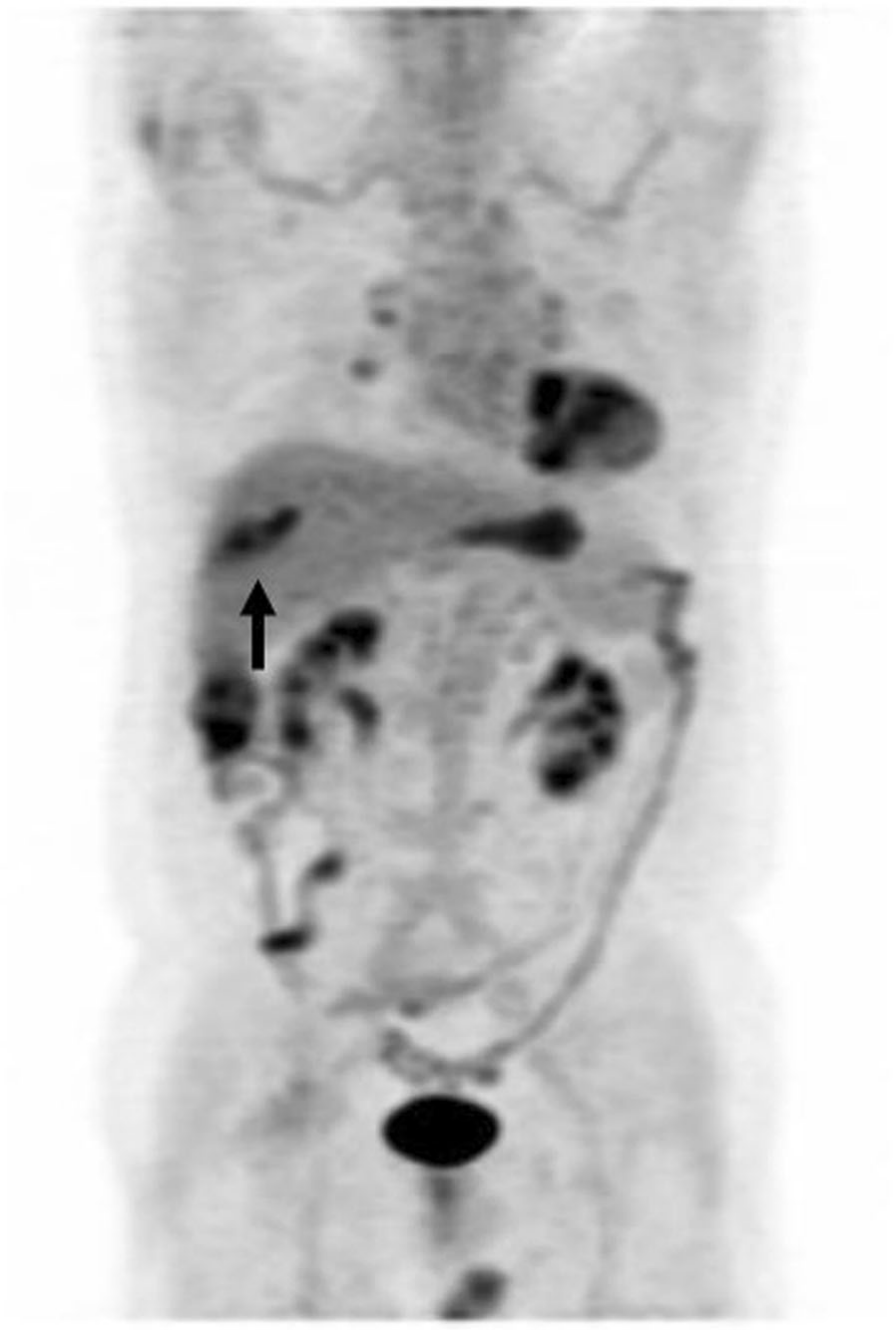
PET imaging revealed abnormal uptake in S5 that was consistent with the site of bile duct dilatation, as observed from CT/MRI findings (arrow)
